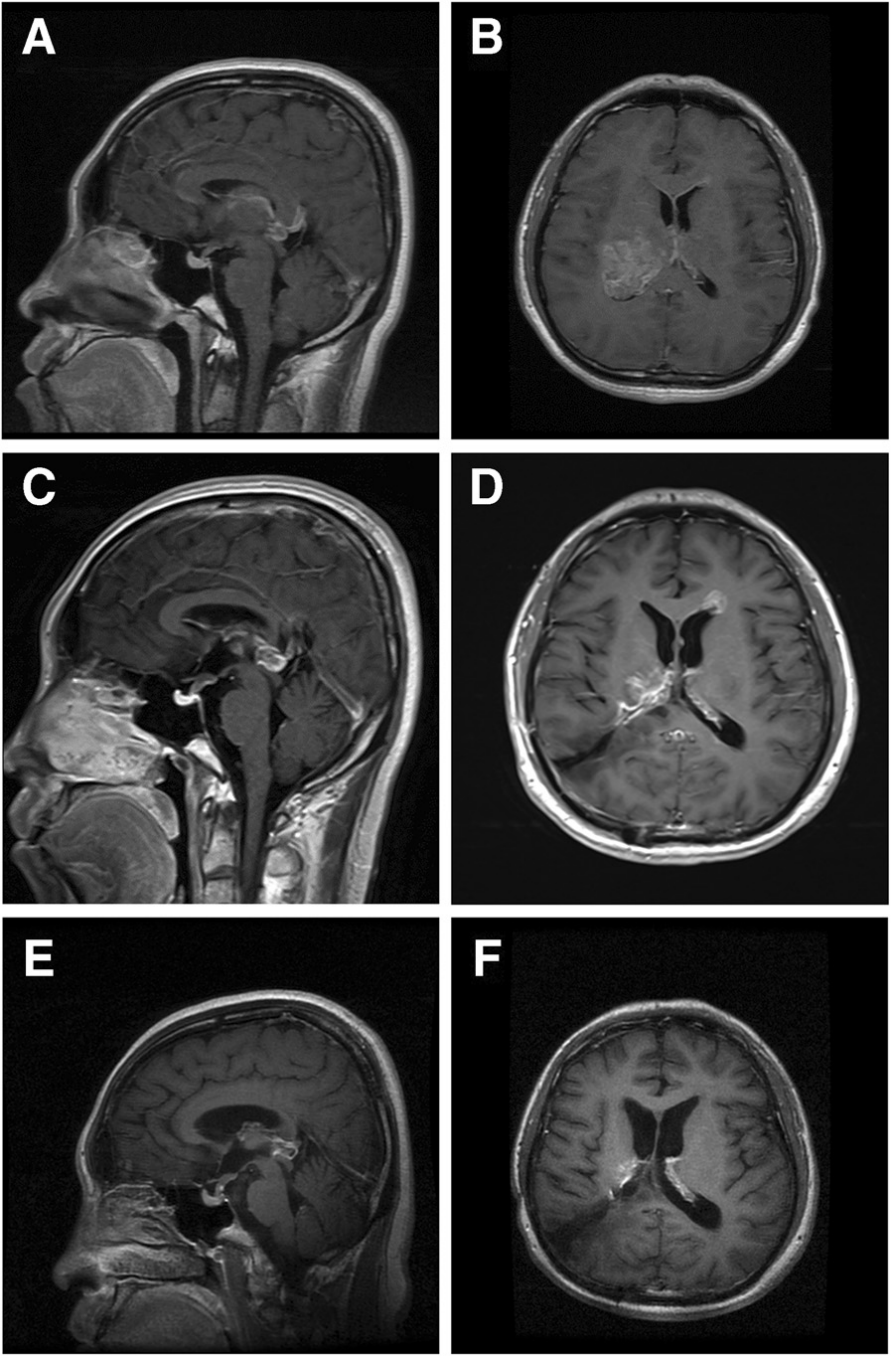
The axial and sagittal contrast magnetic resonance imaging (MRI) results of the patient. At primary diagnosis, there were enhancements of the right thalamus, anterior angle of left ventricle and pineal region (A, B). After the operation, the lesion of the right thalamus was completely removed (C, D). Lesions on the left ventricle and pineal region were eliminated after 12-cycle administration of temozolomide (E, F).
